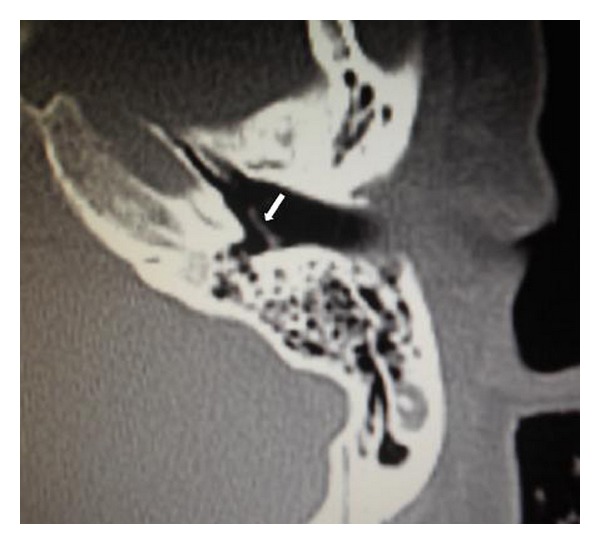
Axial computed tomography scan of the left temporal bone showed thickening of the tympanic membrane with a normal middle ear and mastoid cavity (arrow: tympanic membrane).
